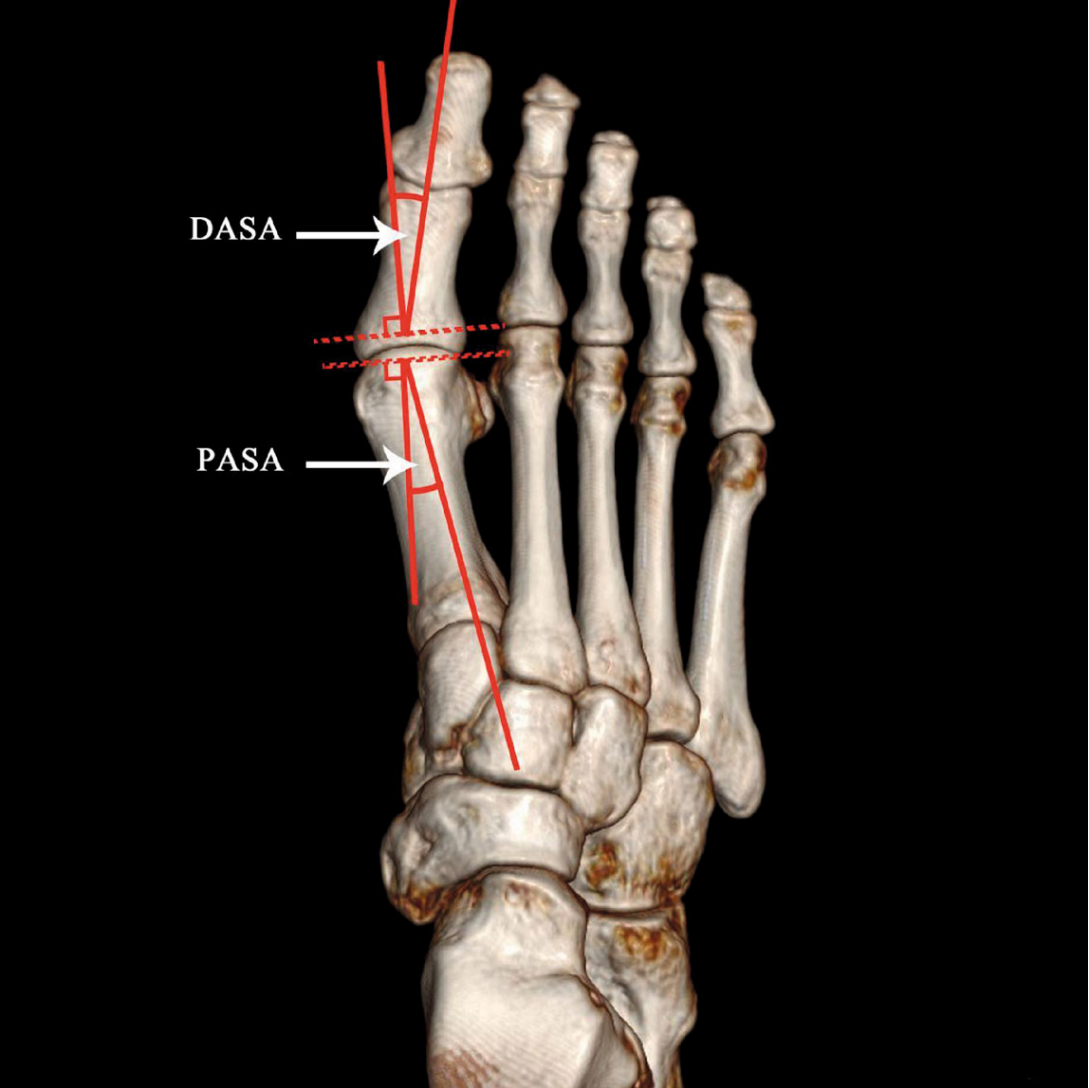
The angle to assist in judging the severity of HV. Distal articular set angle (DASA): on orthographic X-rays, the angle between the vertical line of the basal joint surface of the first phalangeal bone and the long axis of the first phalangeal bone, normal < 7°. Proximal articular set angle (PASA): on the orthographic X-ray film, the angle between the vertical line of the first metatarsal joint and the first metatarsal axis, normal < 10°.
